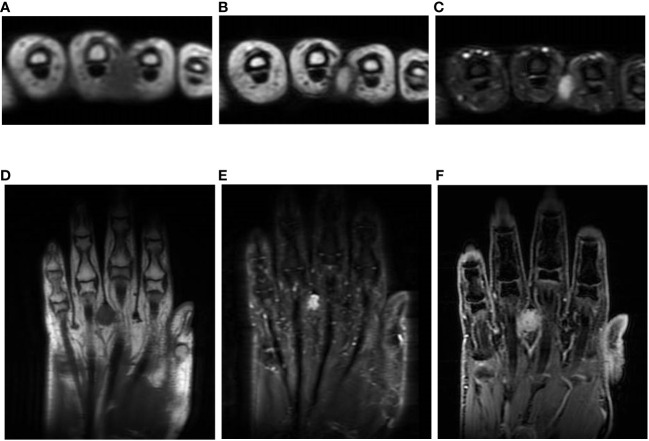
Imaging findings. T1-weighted axial images showing low signals (A, D); homogeneous high signal on T2-weighted images (B) and short tau inversion recovery images (C, E); and axial and central enhancement on a gadolinium-enhanced T1-weighted image (F).
